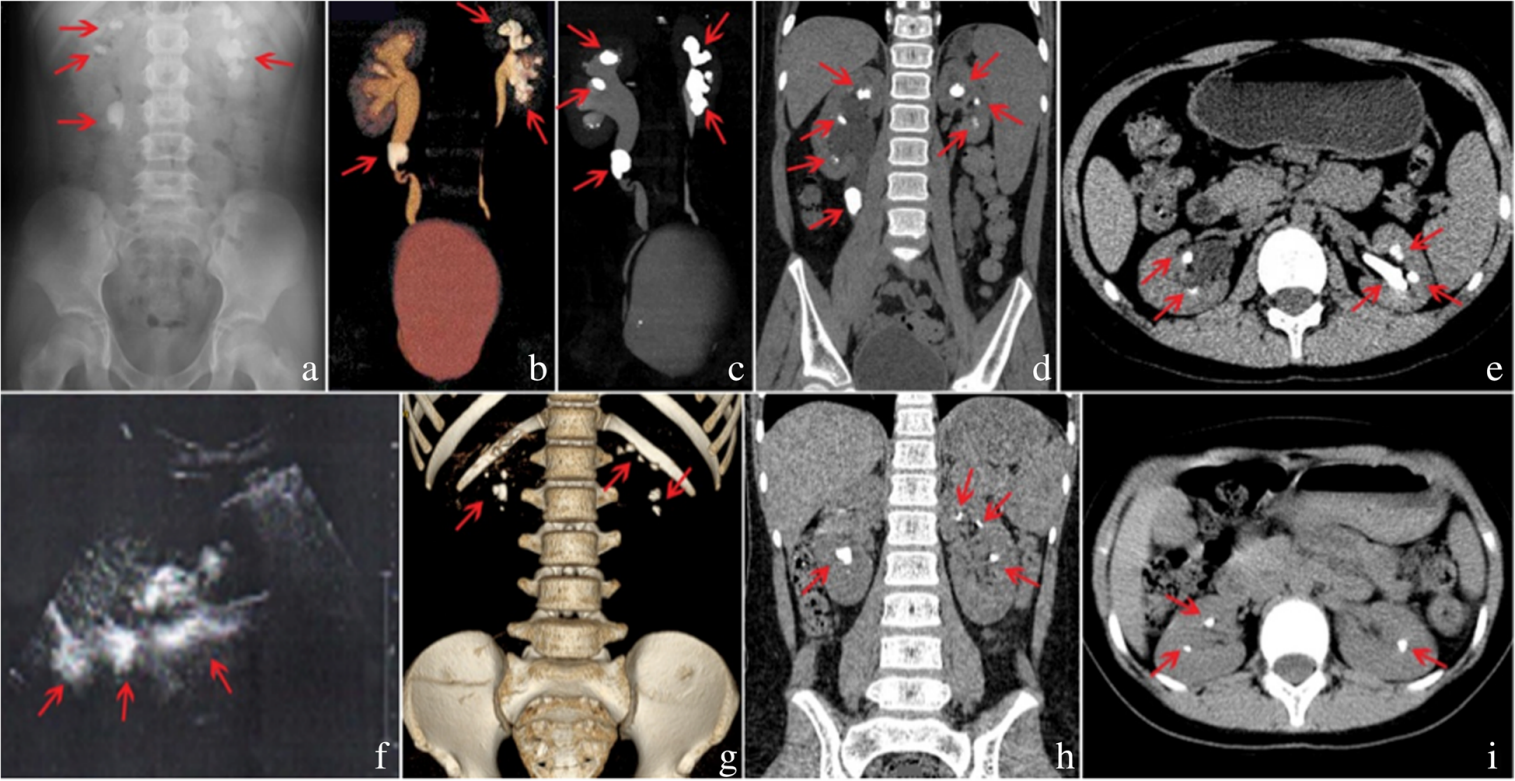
Radiographic findings in patient 1 (a-e) and patient 2 (f-i). Arrows indicate stones. a: abdominal X-ray, b: CT (coronal image), c: VR (volume rendering), d: MIP (maximum intensity projection), e: CT (axial image), f: ultrasound, g: VR (volume rendering), h: CT (coronal image), i: CT (axial image).
